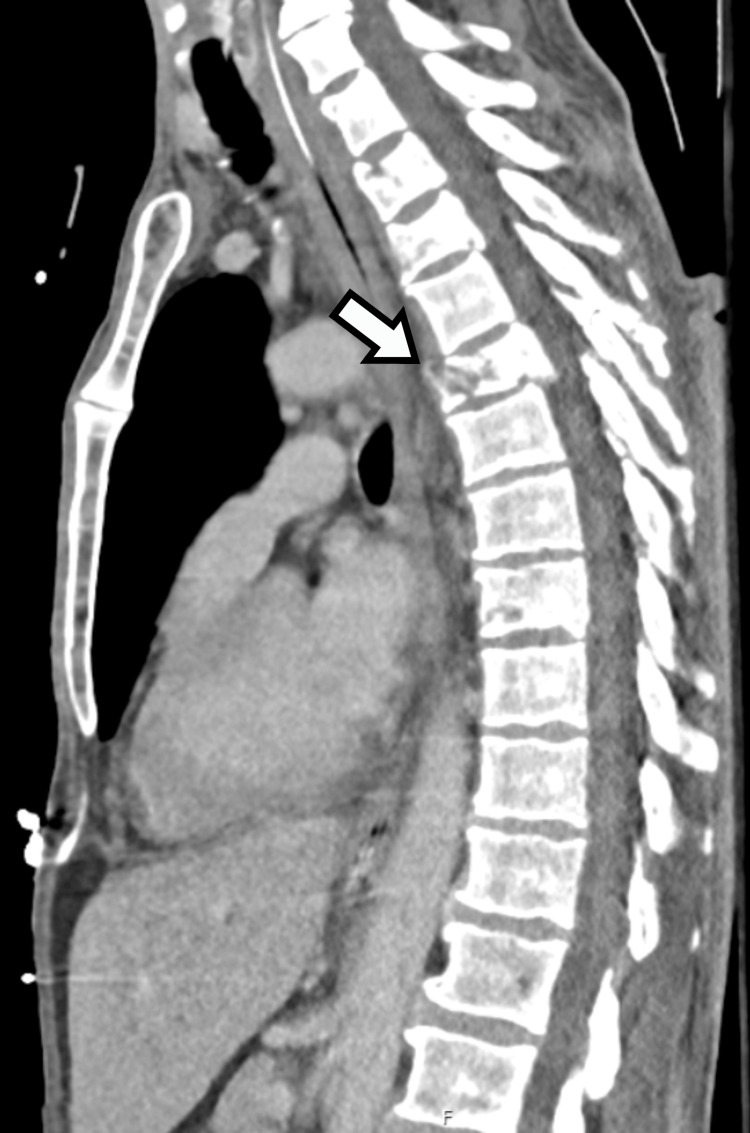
Spinal CT scan in the ER Arrow: T5 with a complete burst fracture. CT: Computerized tomography; ER: Emergency room
